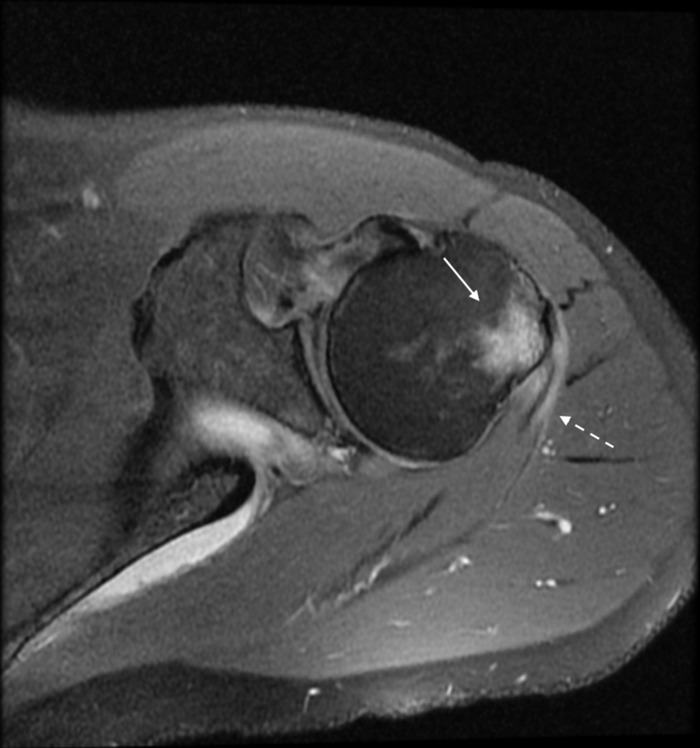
Axial proton density weighted magnetic resonance imaging of the left shoulder with fat saturation again demonstrates edema signal within the greater tubercle (arrow). Edema is also deep in the deltoid muscle and within the teres minor tendinous insertion (dashed arrow).
